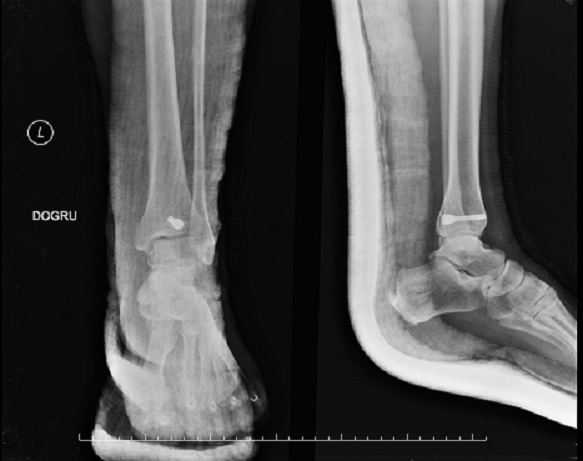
Closed reduction and One compression screw was fasted at posteroanterior orientation and the fracture was fixed. Anteroposterior (a) and lateral X-ray of the ankle
